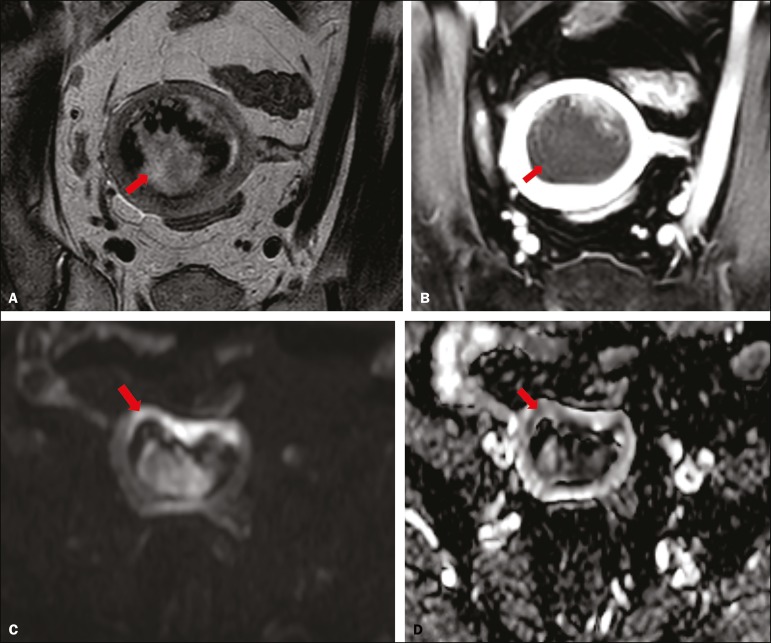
MRI of a 66-year-old woman with endometrial cancer. A: Axial
